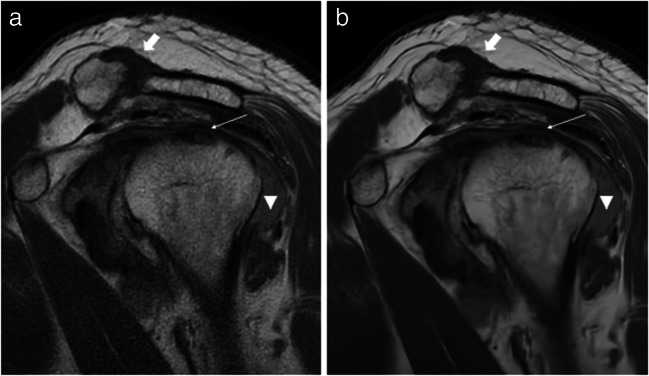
MR images of the right shoulder joint of a 78-year-old male with chronic shoulder pain. a Conventional PROPELLER sagittal oblique T1-weighted (T1w) image, b sagittal oblique T1w image after post-processing using DL images shows degenerative changes of the acromioclavicular joint (broad white arrow), subchondral cysts in the humeral head (thin white arrow), and a joint effusion (triangle). All pathologies can be delineated in both sequences; however, the post-processed sequence is less noisy so the pathologies can be identified easily
